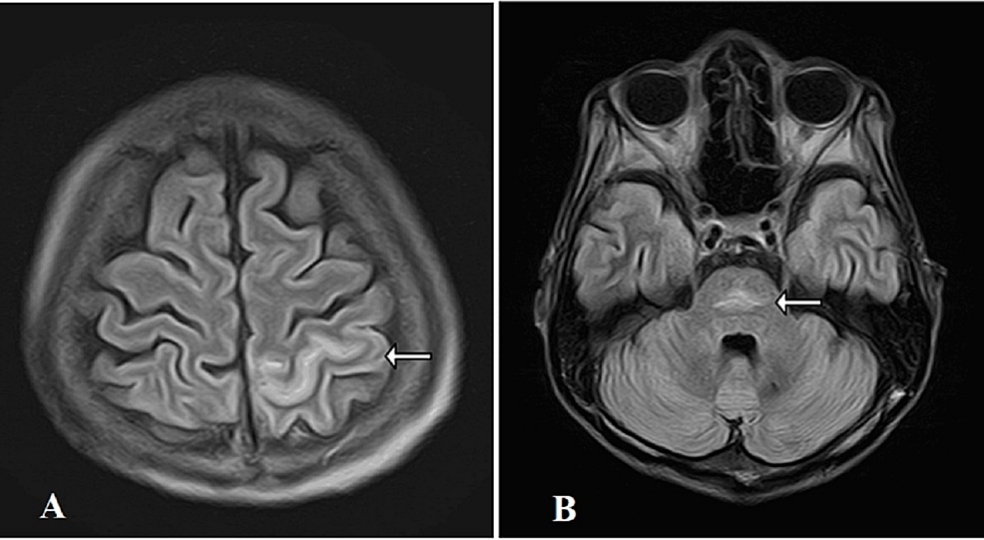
FLAIR MRI of the head On hospitalization day 14, head magnetic resonance imaging (MRI) in a fluid-attenuated inversion recovery (FLAIR) sequence showed a high signal area in the left parietal lobe (Figure 4A). On hospitalization day 44, head MRI on FLAIR showed high signal area in the pons (Figure 4B).
